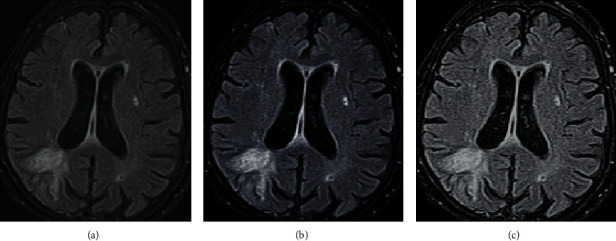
Results of denoising of MRI images of cerebral stroke patients by each algorithm. (a) The original image. (b) The results of denoising by NLM algorithm. (c) The results of denoising by low-rank matrix algorithm.
